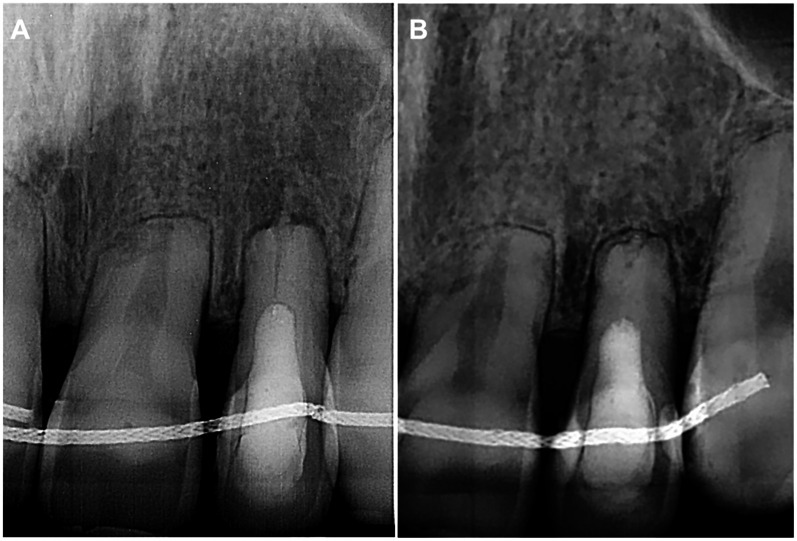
(A) Three-month follow-up periapical radiograph revealing a completely healed periapical lesion on tooth 22. (B) Four-year follow-up periapical radiograph demonstrating no signs of periapical pathology.
